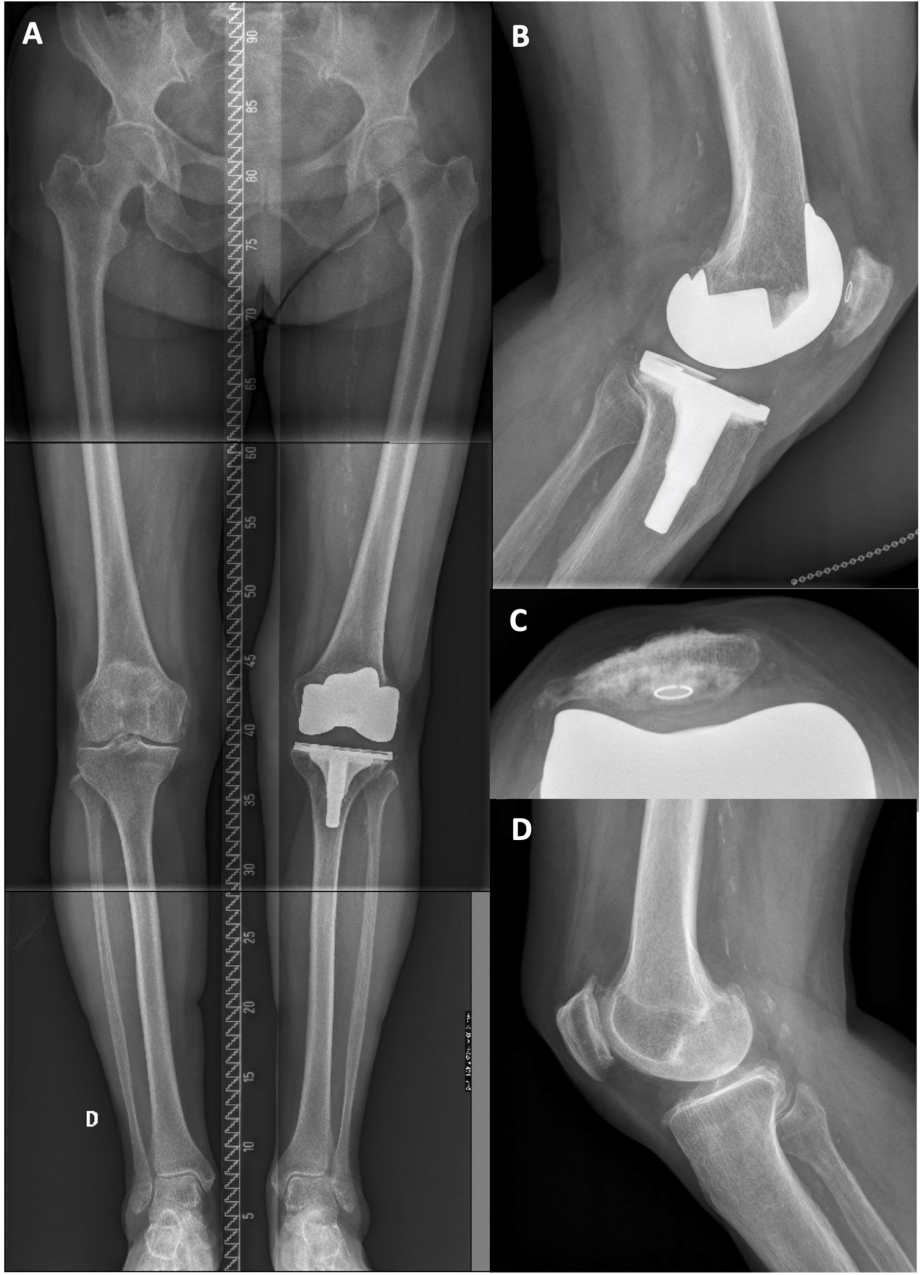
(A) Pre-revision long leg standing X-Ray reveals a left implant mDFA of 2° varus and an mPTA of 2° valgus (0° varus aHKA). On the intact right side, the mDFA is 2.0° valgus and mPTA 3.5° varus (aHKA of 1.5° varus). (B) Left knee lateral view where the implant posterior tibial slope is 12.0°. (C) Left patella skyline view showing a lateral patellar retinaculum calcification and a well-centered resurfaced patella. (D) Right knee lateral view where the native tibial slope is 6.0° posterior.
